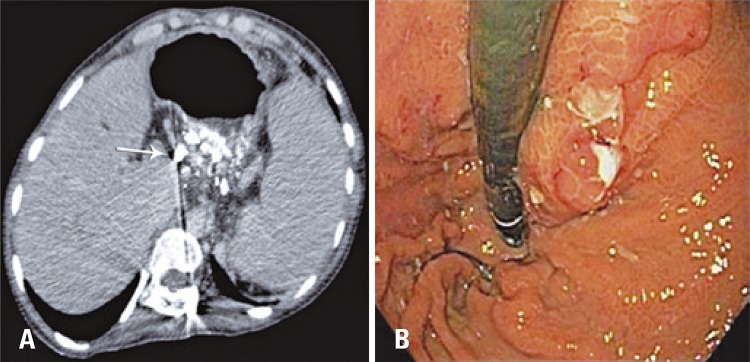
Control exam after 30 days. (AG) Computed tomography after 30 days showing gastric varices filled with fluid agent (Onix®), white arrow. (B) Upper digestive endoscopy of control 30 days after the procedure
